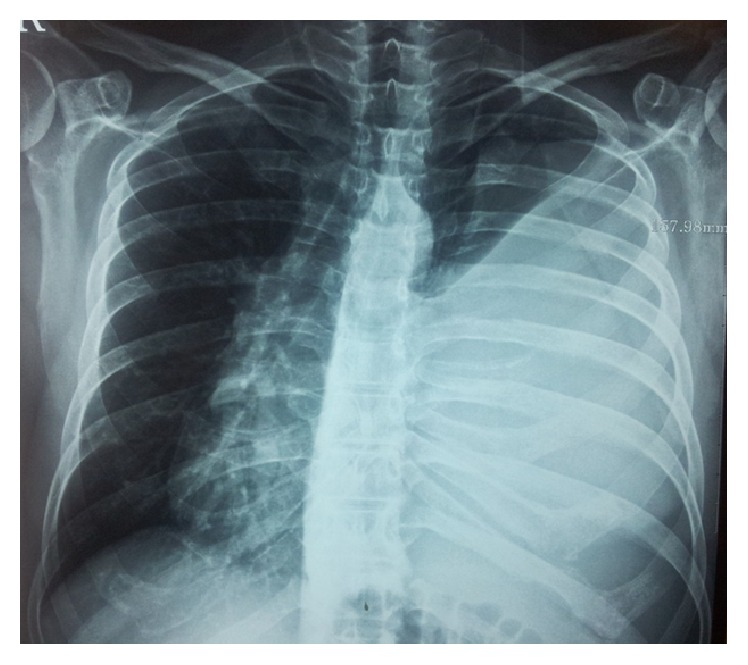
Chest X-ray shows a large left-sided mass density with obvious mediastinal shift including trachea and heart displacement.
